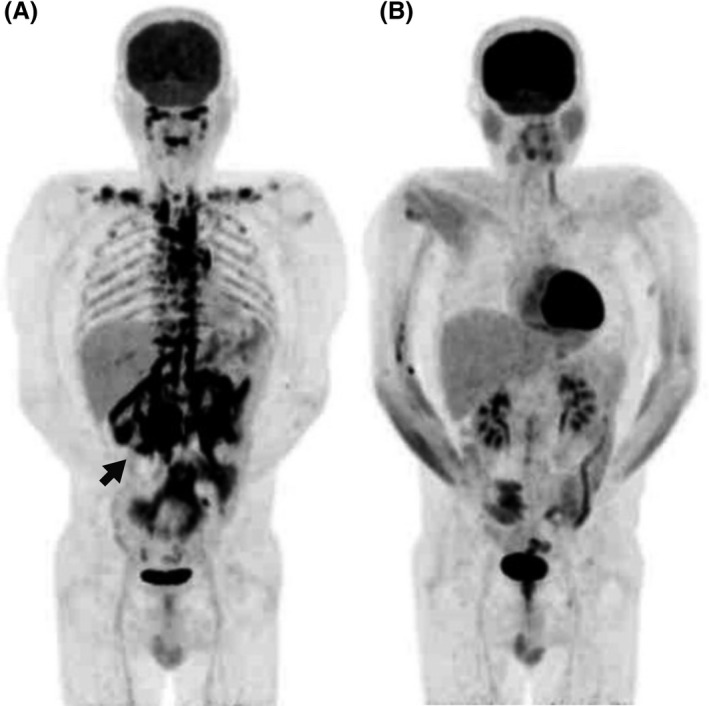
Planar view of ^18^F‐fluorodeoxyglucose (FDG) positron emission tomography. A, Multiple high accumulations of FDG not only in the retroperitoneal tumor (arrow) but also in the peritracheal, upper mediastinal, supraclavicular, perispinal, periaortic, and perirenal spaces; B, disappearance of multiple FDG accumulations 1 mo after the resection of the right retroperitoneal paraganglioma
